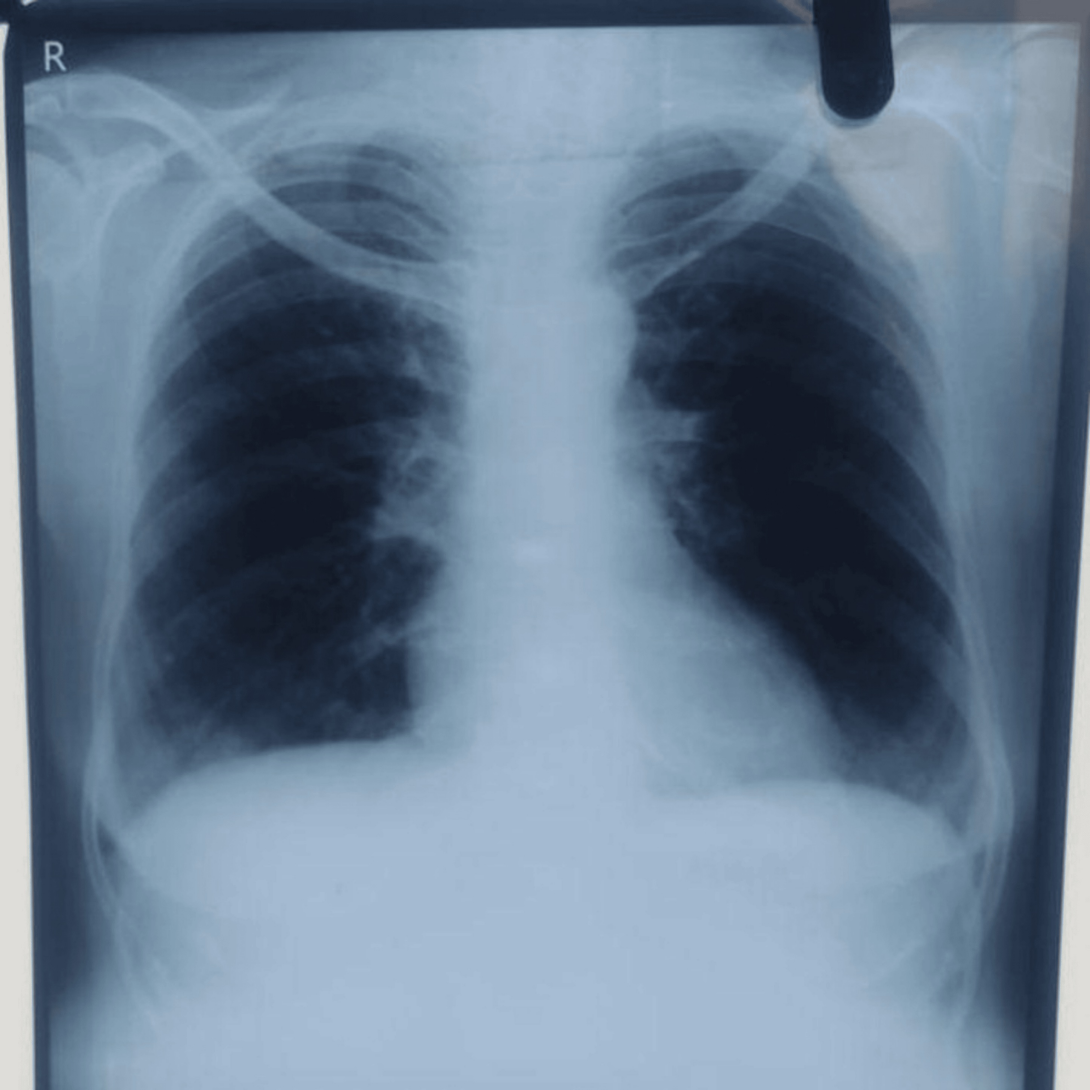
Normal chest X-ray posteroanterior (PA) view
